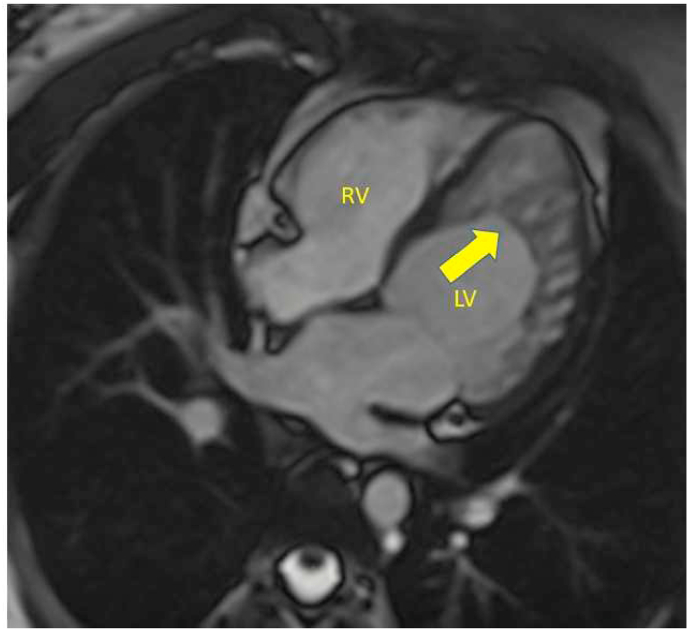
Cardiac magnetic resonance imaging steady-state free precession still-frame 4-chamber view showing left ventricle non-compaction (arrow). LV left ventricle, RV right ventricle.
